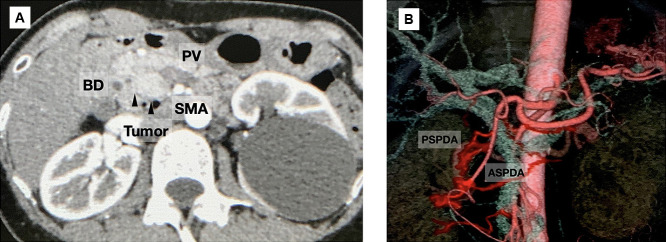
Preoperative images of Patient 5 (A) Preoperative enhanced CT showing a 17-mm well-enhanced tumor (black arrows) in the pancreatic head. (B) CT angiography showing the preoperative arterial anatomy. CT: computed tomography, PV: portal vein, BD: bile duct, SMA: superior mesenteric artery, PSPDA: posterior superior pancreaticoduodenal artery, ASPDA: anterior superior pancreaticoduodenal artery
